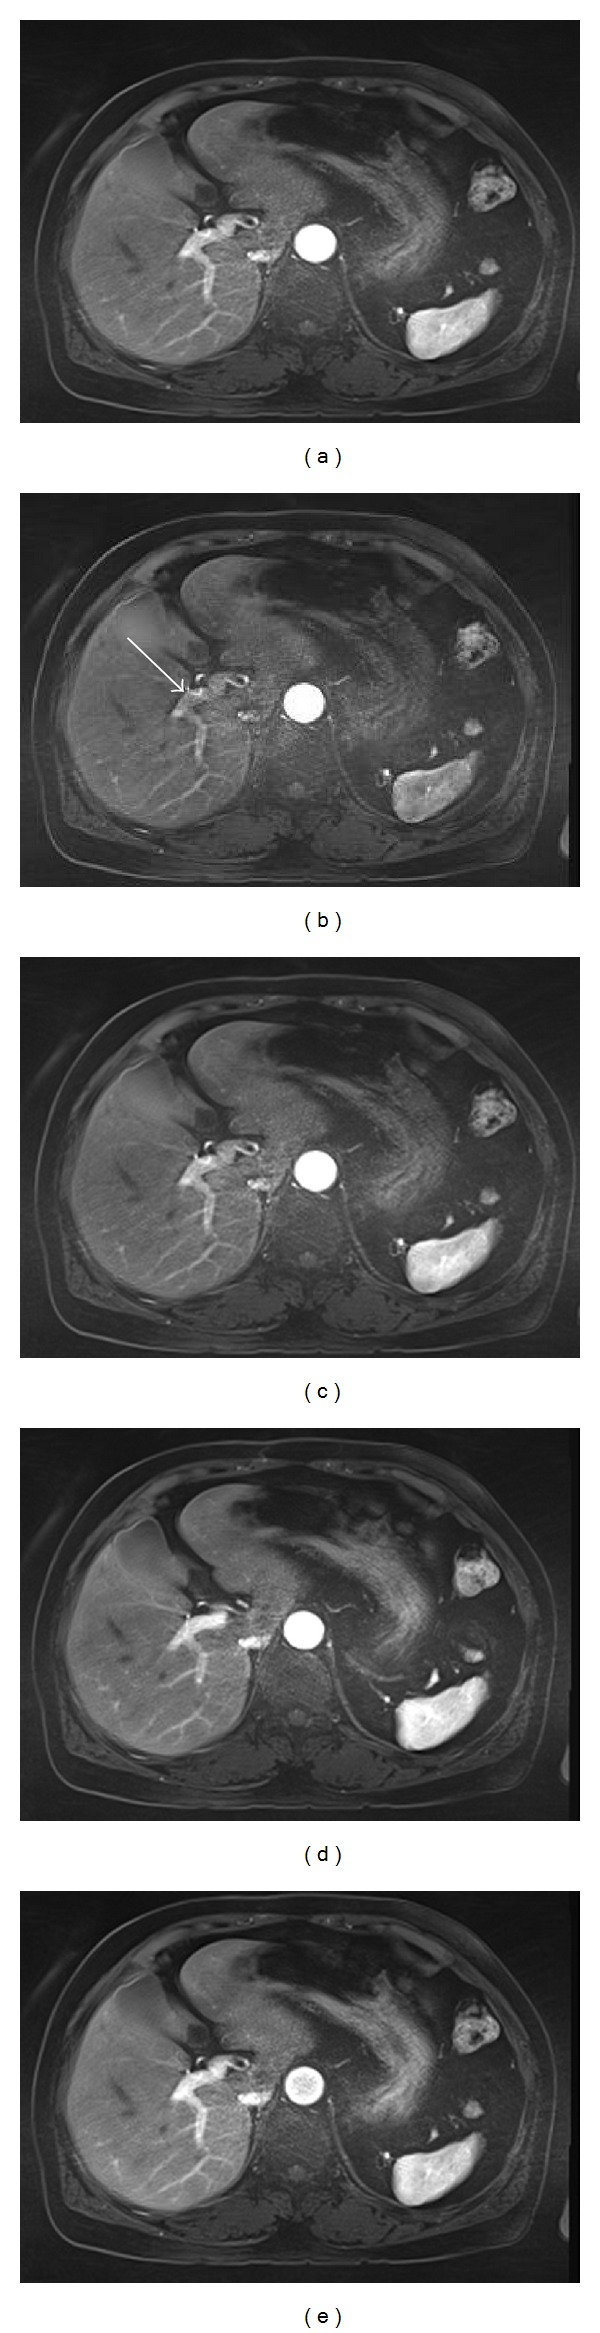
MR images obtained in a 68-year-old woman for workup of metastasis. Contrast-enhanced dynamic 3D radial GRE using KWIC during hepatic arterial dominant phase imaging comprised four subframe images. The full-frame 3D radial GRE (a) image shows homogenous enhancement in the portal vein, but not in the hepatic vein. The first subframe image (b) obtained at 5-second temporal resolution demonstrates minimal portal venous enhancement with laminar flow (arrow). On the next serial three subframe images ((c)–(e)), the portal vein and hepatic parenchyma have gradually increasing enhancement.
